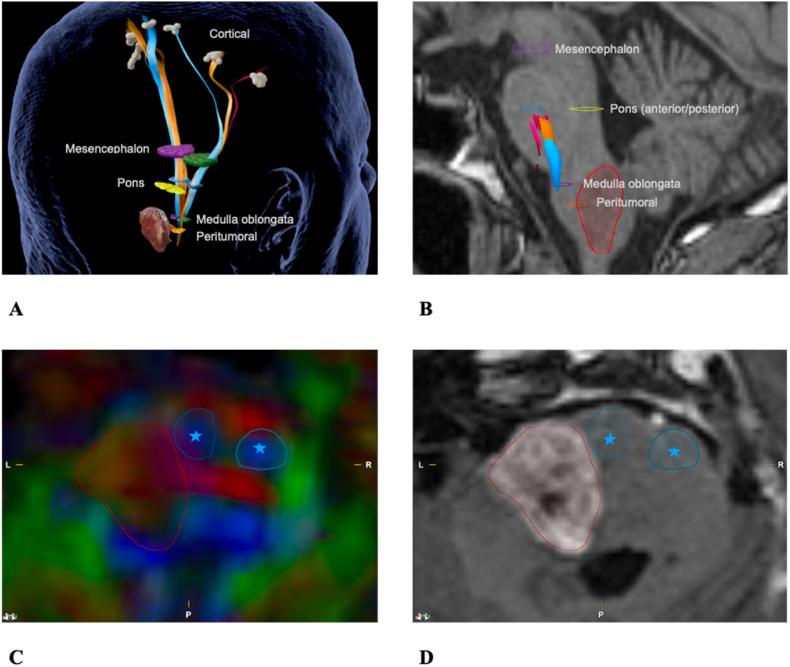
ROI setting. A: Overview of all ROIs, the descending motor fiber tracts and the tumor (patient #7). Color code: Blue for leg (CST), Orange for hand (CST) and Pink for face (CBT) fibers. B: Sagittal T1 (patient #7): overview of all ROIs in the right hemisphere; red boundaries mark the tumor. C-D: Patient #12 example of segmental ROI setting. The ROIs (blue stars) are in the anterior Pons and the borders of the tumor (marked in red); MRI sequences: 2C – FA map, 2 D – T1-weighted MP-RAGE
